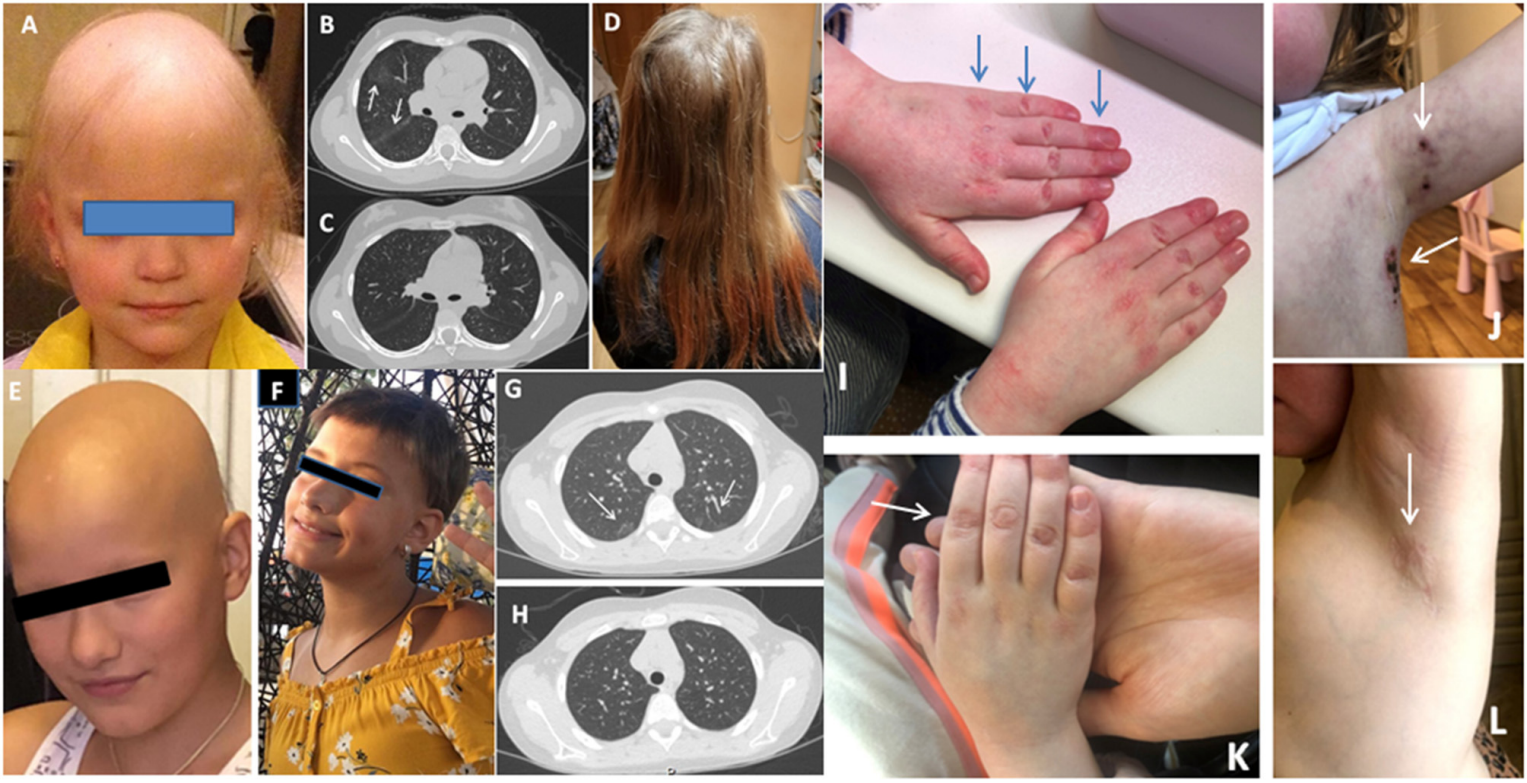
(A) Alopecia in JIA2 patient (before tofacitinib); (B) Chest CT from patient JIA2 (interstitial thickness and ground-glass opacities - arrows); (C) Chest CT from patient JIA2 following 3 years of tofacitinib therapy (resolution of interstitial thickness and ground glass opacities); (D) Hair growth in JIA2 patient (3years on tofacitinib); (E) Alopecia in patient JIA10 before tofacitinib treatment and after 6 months of treatment (F); (G) Ground-glass opacities in patient JDM1 before tofacitinib (arrows) and 2 years on tofacitinib (H); (I) The hands with Gottron papules before tofacitinib treatment in JDM2 patient and the left axillary area (arrows) with necrotizing changes (J); (K) the right hand 16 weeks after tofacitinib treatment and the left axillary area (L) after 6 months of tofacitinib treatment of the same patient.
